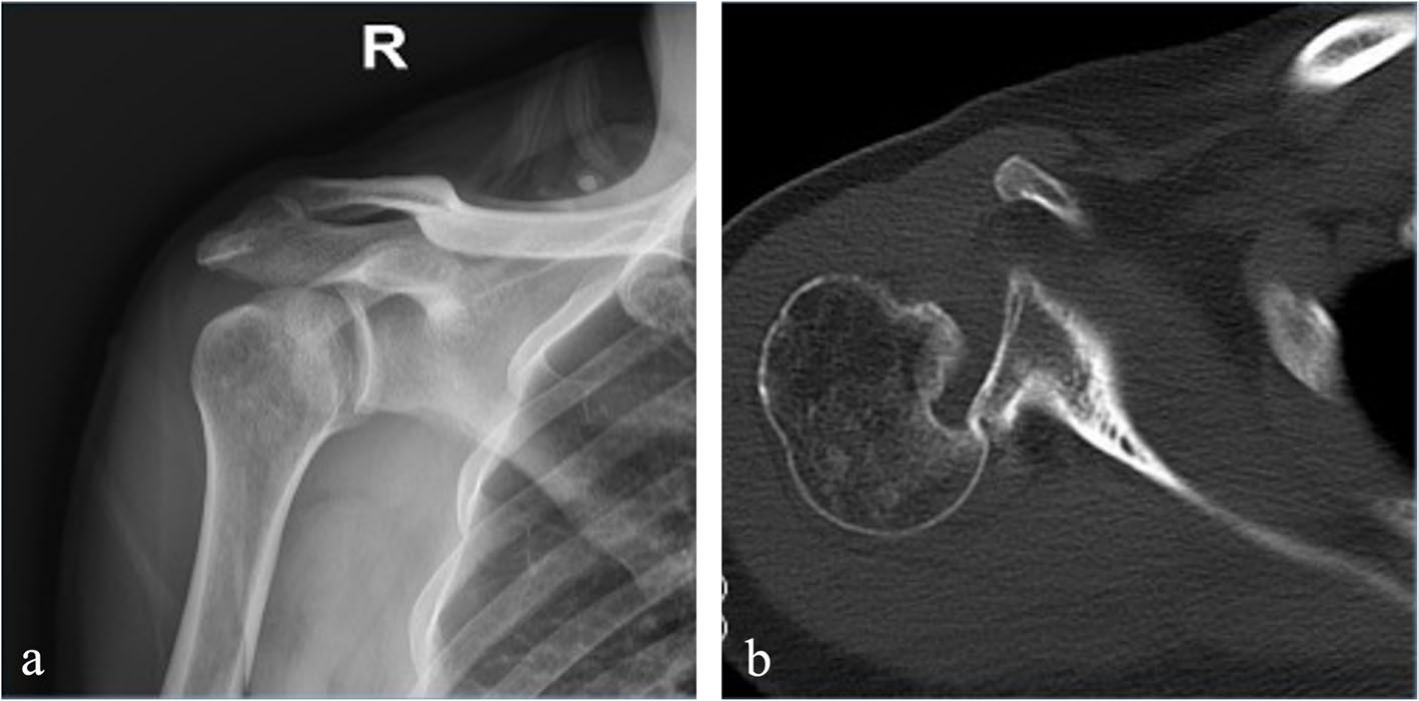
Preoperative radiographs of the right shoulder of a patient with a 8-week delay from injury to diagnosis showing a chronic locked posterior dislocation of the shoulder with a large reverse Hill-Sachs lesion
